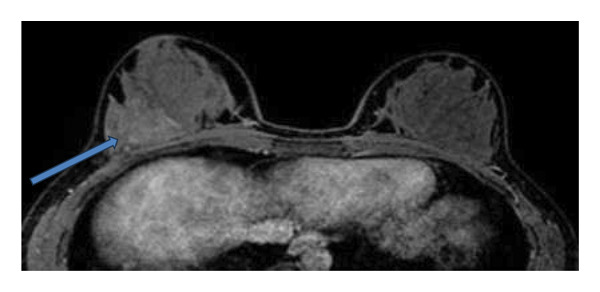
The same patient as in Figure 13. Post‐contrast MRI sequences demonstrate a non‐mass‐like regional area of weak and delayed enhancement in the lower outer quadrant of the right breast (arrow).
